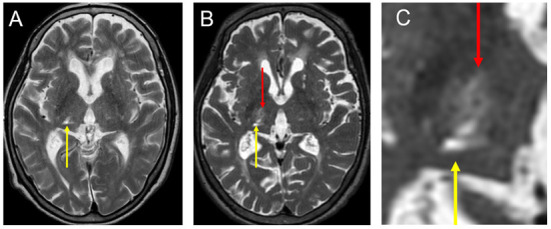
Preoperative images of her MRI. (A) T2 image taking before FUS—thalamotomy after right thalamic infarction (yellow arrow). The infarction was located in the right VC extending to the pulvinar nucleus. (B) T2 image taking a day after FUS—thalamotomy. Note that the lesion with super low intensity with surrounding high intensity was located in the Vim (red arrow). (C) High magnification of B. FUS, focused ultrasound surgery; MRI, magnetic resonance imaging; VC, ventral caudalis nucleus; Vim, ventral intermediate nucleus.
